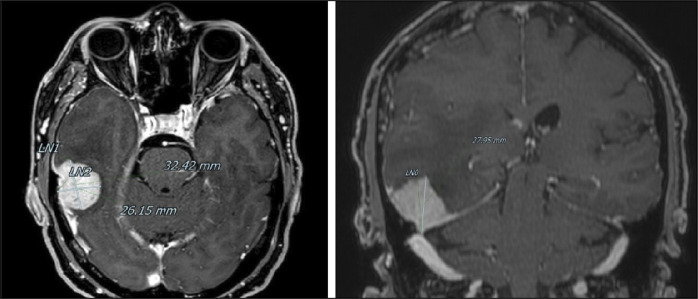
Atypical meningioma in the right temporal lobe at magnetic resonance imaging, axial, and coronal images.
